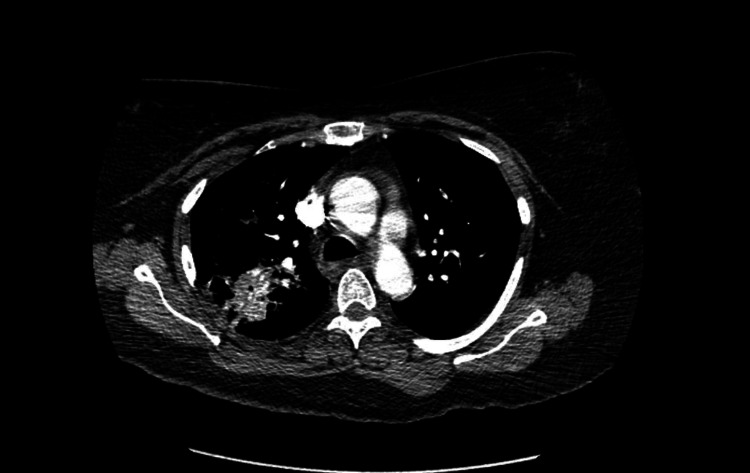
CTA of the chest The impression of the imaging is as follows: Large area of airspace disease within the right upper lobe most likely due to bacterial pneumonia Probable second area of pneumonia within the right lower lobe. The left lung is grossly clear other than some slight atelectasis. No evidence for PTE. CTA: Computed tomography angiography; PTE: pulmonary thromboendarterectomy
